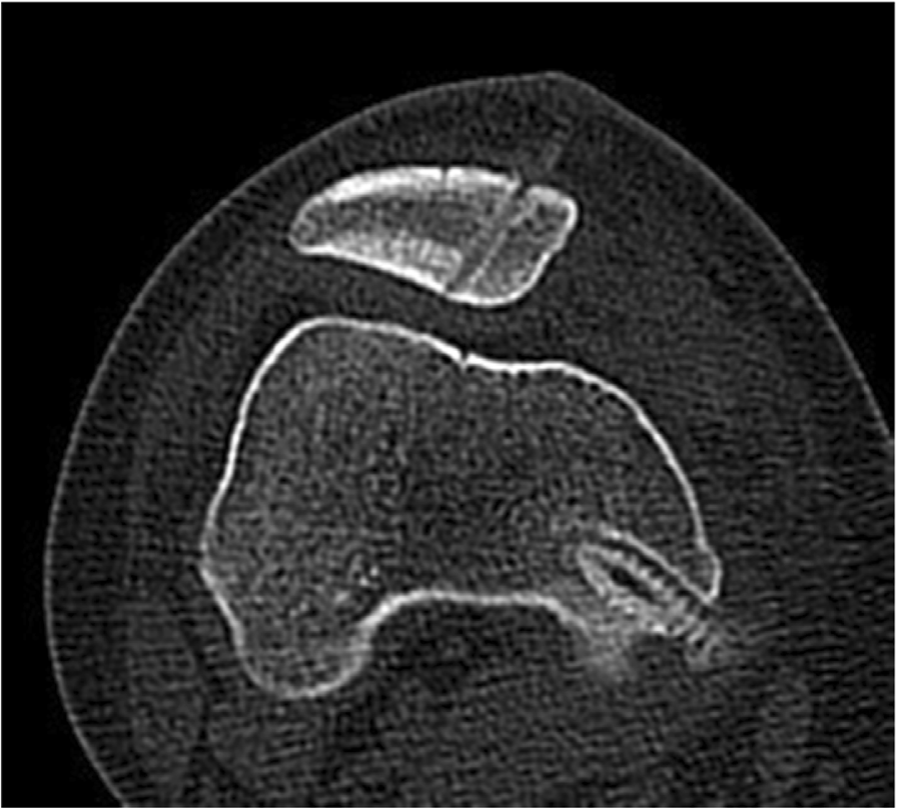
The suture anchor passing through the patella, as shown on CT scans
